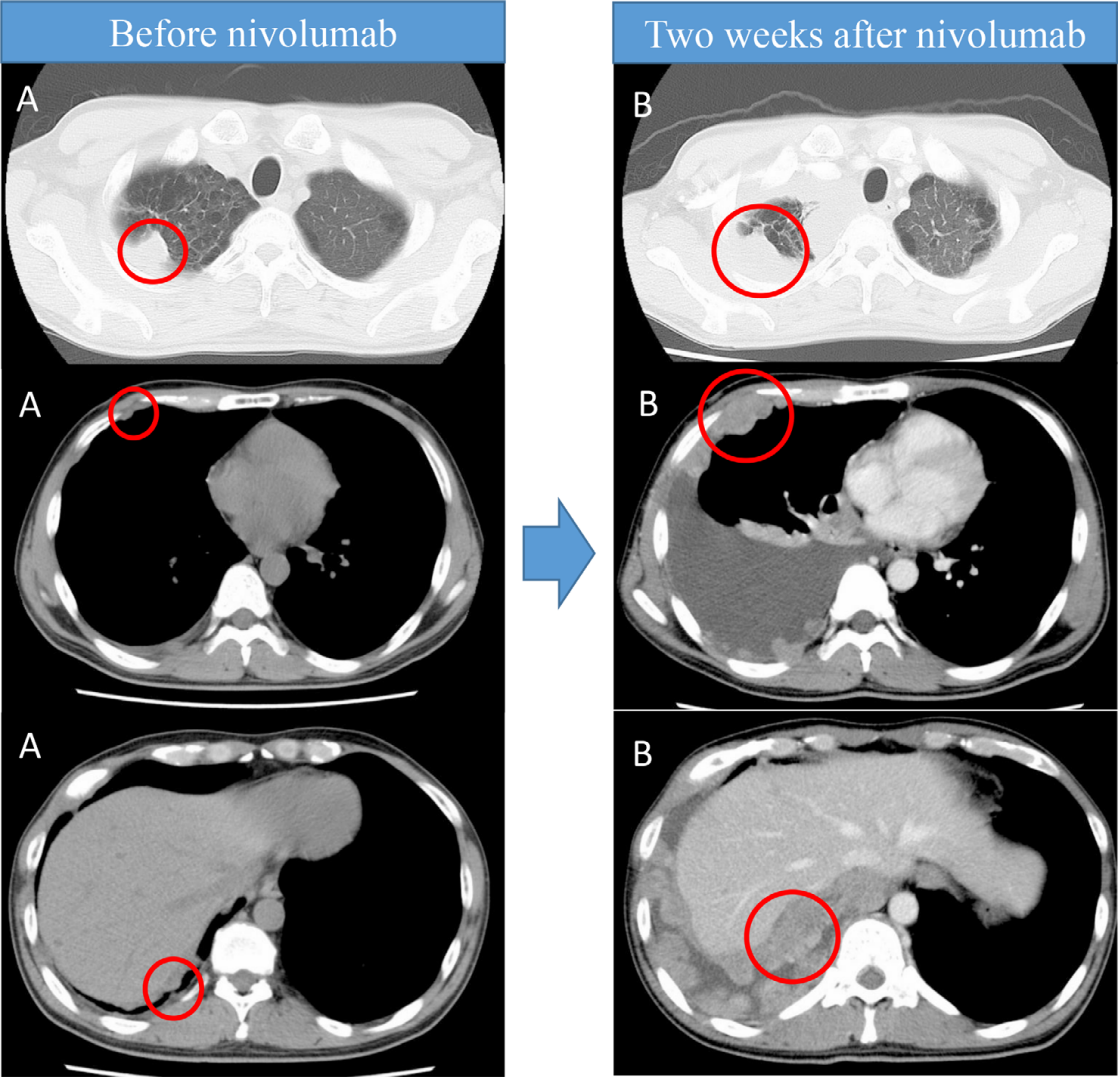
Chest computed tomography (CT) images before nivolumab treatment (A) and two weeks after nivolumab treatment (B), showing increased rapid pleural effusion and dissemination.
